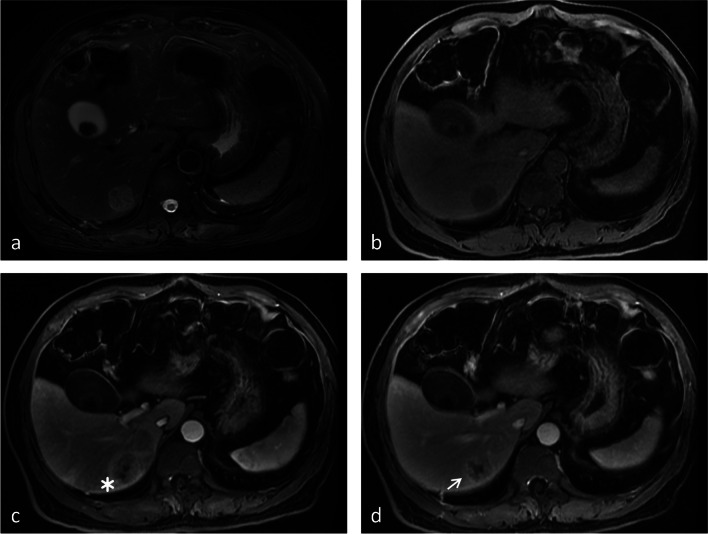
Image from a 76-year-old male with CRLM. a The T2-weighted image and (b) T1-weighted image show a 2.6-cm lesion in segment VI of the liver. c The arterial phase image shows peripheral hepatic enhancement (star). d The portal venous phase image shows continuous rim enhancement (white arrow). Hepatic capsular retraction, vessel penetrating the tumor, and upper abdominal lymphadenopathy are not shown. Thus, a score of 4 was assigned for this patient
